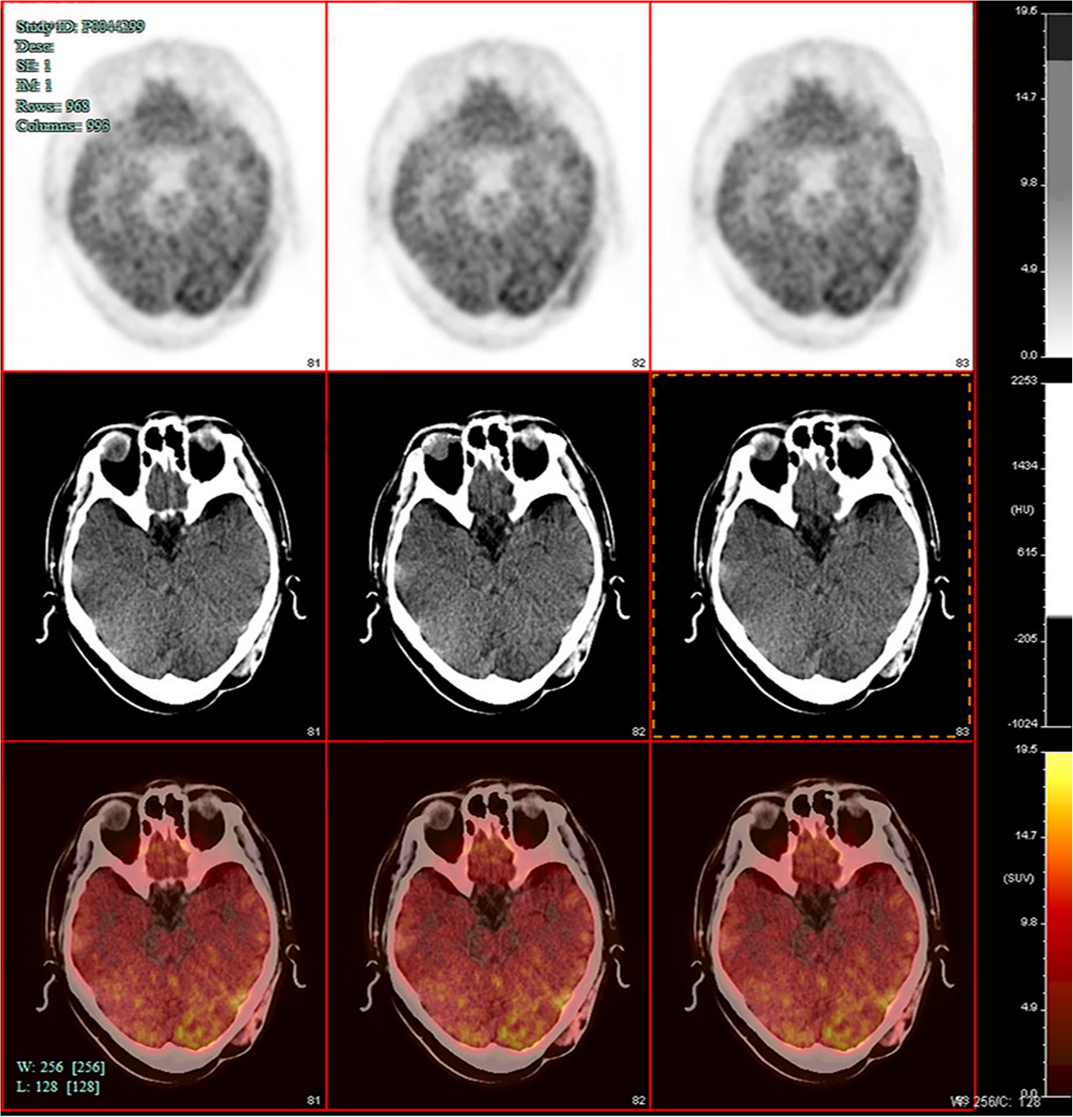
Left occipital poorly differentiated carcinoma after secondary resection: left occipital are scalp and subcutaneous soft tissue density irregularity, with left occipital bone destruction, FDG metabolism increased, consider tumor recurrence
